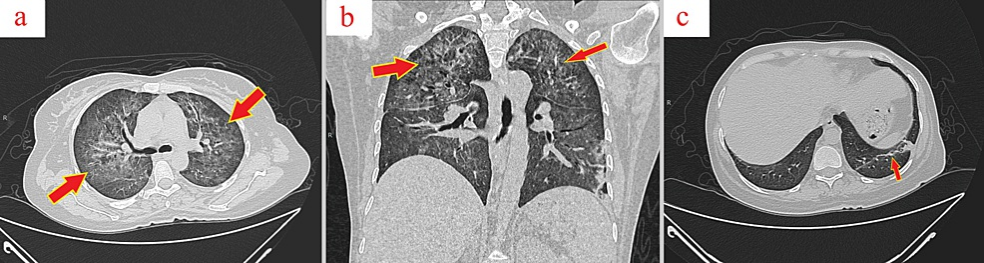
Chest CT scan Chest CT scan reveals confluent ground-glass opacities within both lungs, predominantly in the upper lobes (Figure 2a, 2b) (arrows). A linear atelectatic band is evident in the left lower lobe (Figure 2c) (arrow). These findings are consistent with diffuse alveolar hemorrhage. CT: computed tomography
